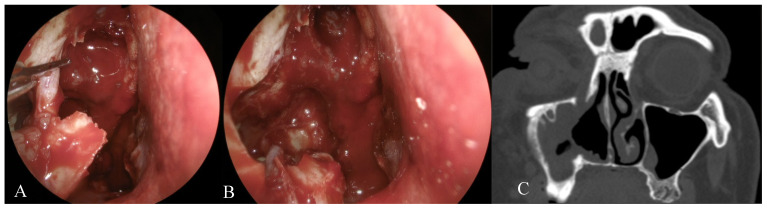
Transection of nasolacrimal duct at the level of orbital floor (A). Nasolacrimal duct removed, no additional procedures aimed at improving tear drainage were performed (B). Postoperative CT of the same patient—amputated nasolacrimal duct on the right side (C).
